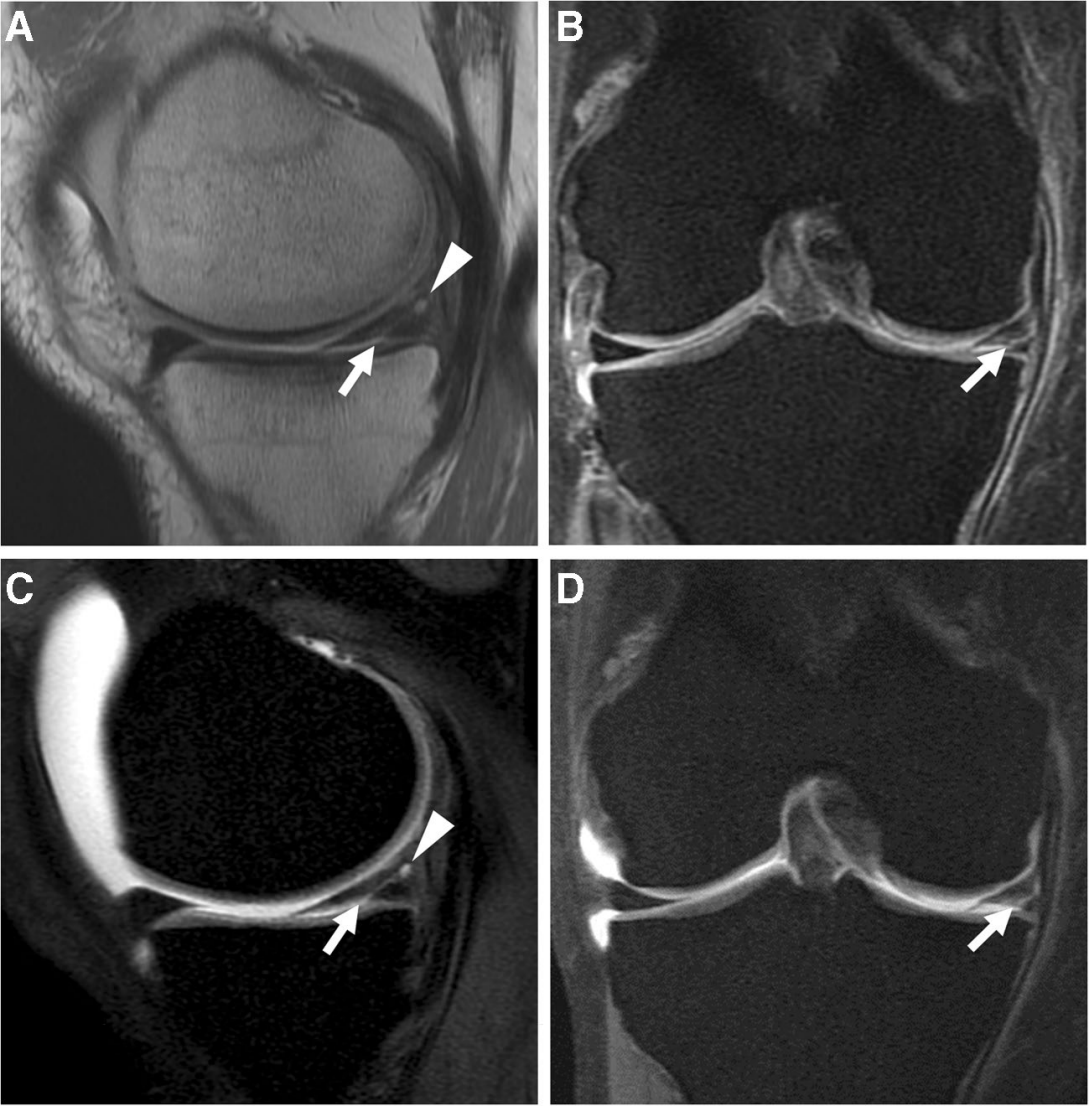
Recurrent meniscal tear. Fifty-one-year-old man with history of meniscal surgery and recurrent symptoms. Sagittal intermediate-weighted (A) and coronal intermediate-weighted fat-suppressed (B) conventional MR images show evidence of partial medial meniscectomy with linear increased signal extending to the inferior surface (arrows) and small posterior parameniscal cyst (arrowhead), consistent with a recurrent tear. Sagittal (C) and coronal (D) T1-weighted fat-suppressed MR arthrogram images demonstrate dilute gadolinium extending through the tear (arrows) and filling the parameniscal cyst (arrowhead)
